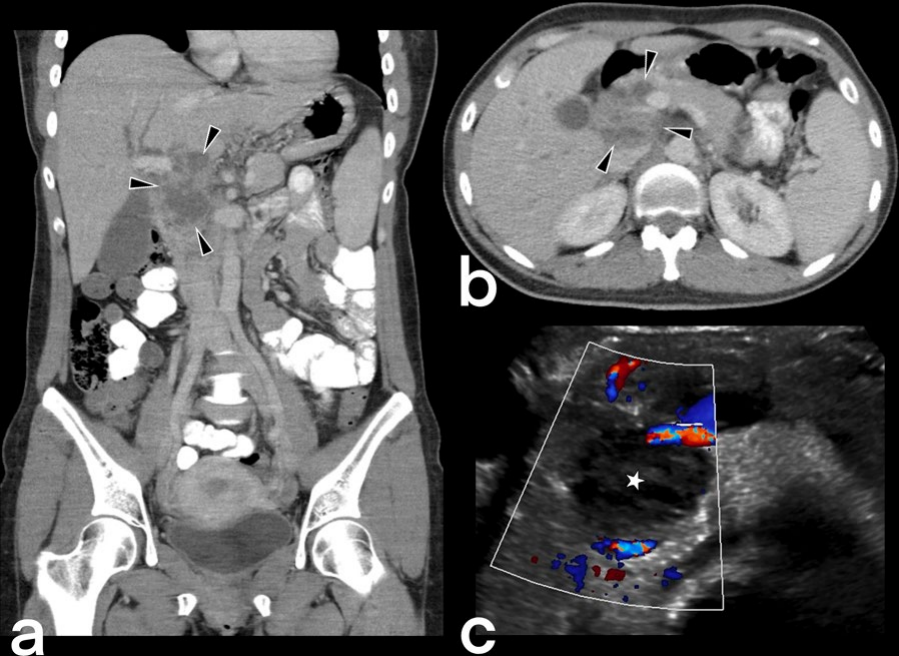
A 40-year-old female. Contrast-enhancement CT scan (a, b) and Doppler ultrasonography (c) demonstrated a large heterogeneous cystic-necrotic mass in the head of the pancreas
